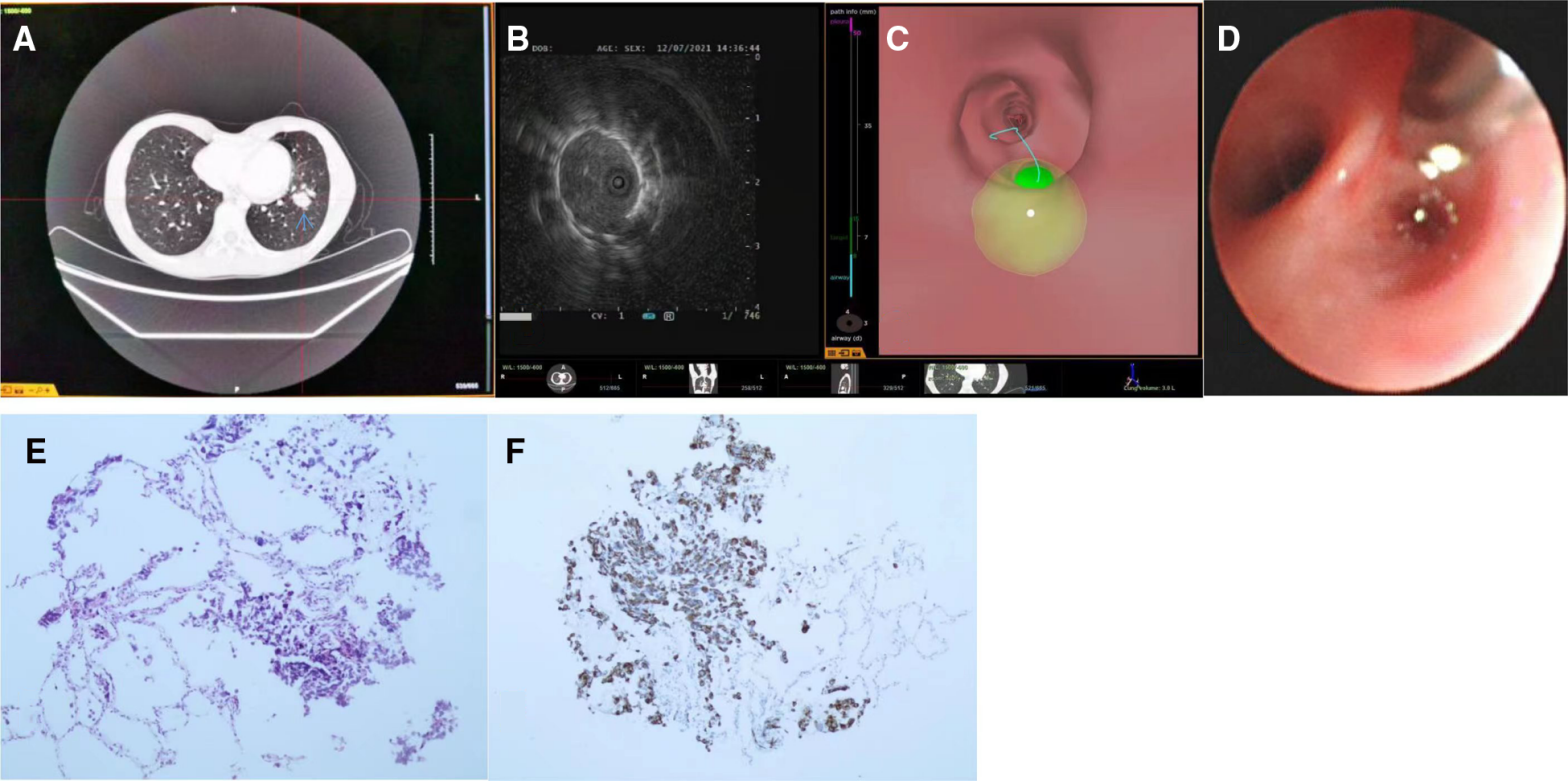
The operation process of archimedes virtual bronchoscopic navigation (VBN)-guided lung biopsy. (A) CT data requirements; (B) Lesions selected and marked (green region); (C) Selected navigation path; (D) Real-time guidance during navigation; (E) Reach the targeted location; (F) Radial endobronchial ultrasound image of the lesion.
